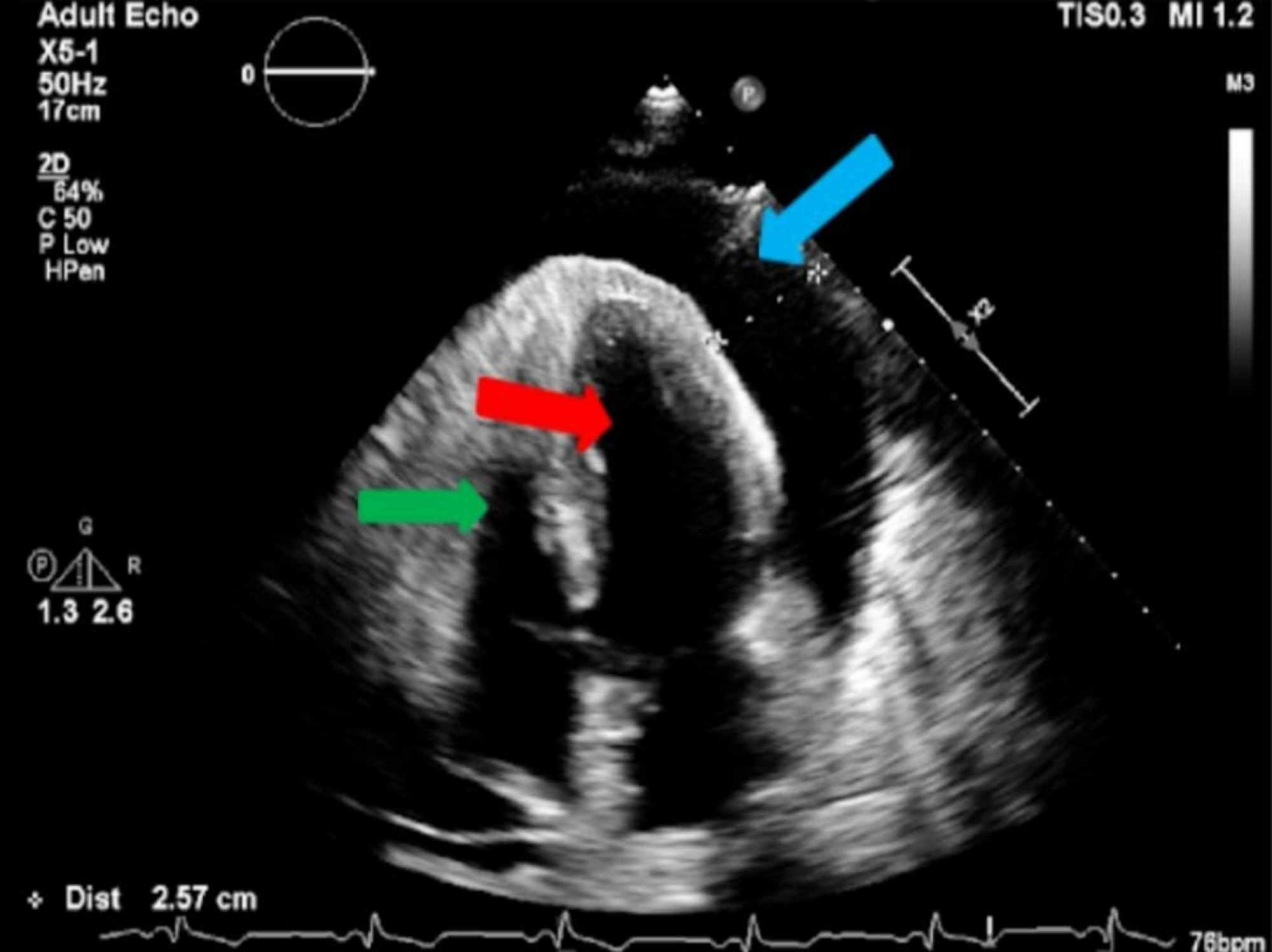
Apical 4 chamber view of a large pericardial effusion measuring 2.57 cm (blue arrow), left ventricle (red arrow), and right ventricle (green arrow).
